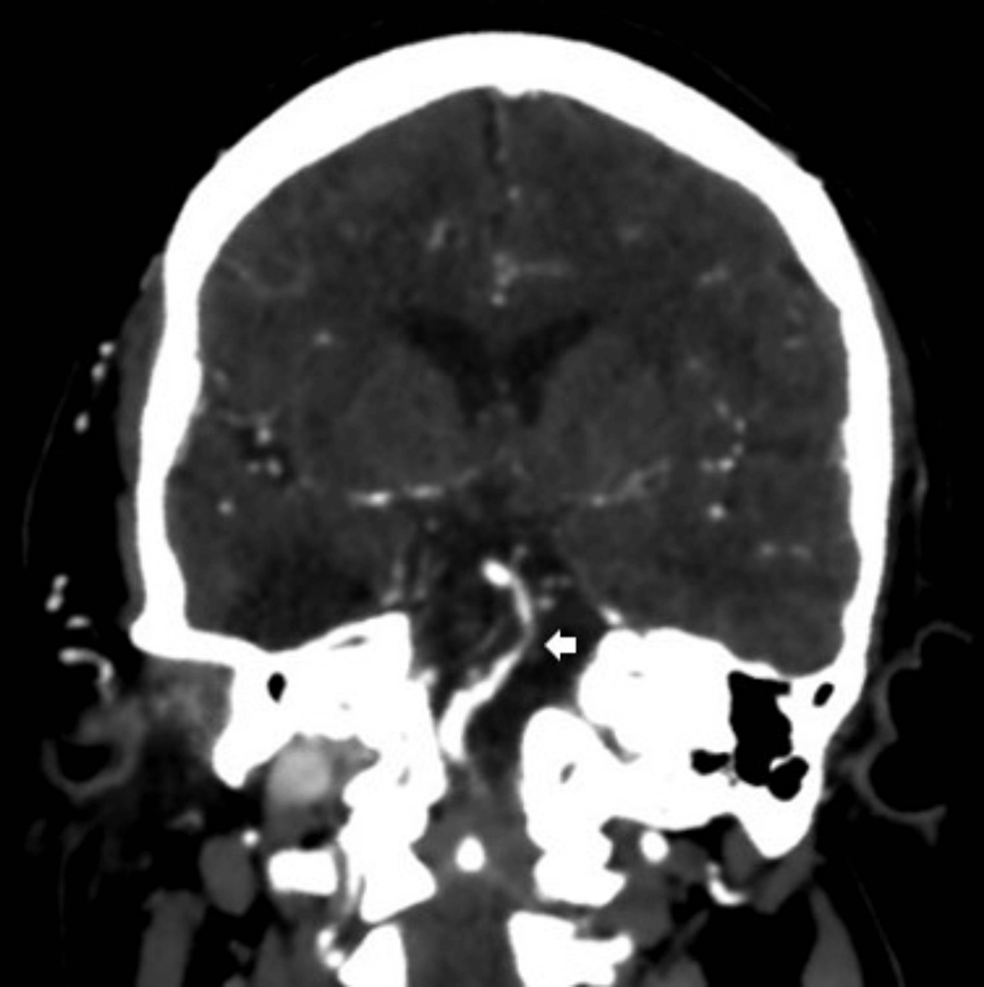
Cerebral CTA in an oblique coronal view showed severe stenosis at the middle part of the basilar artery (white arrow). CTA: computed tomography angiography.
